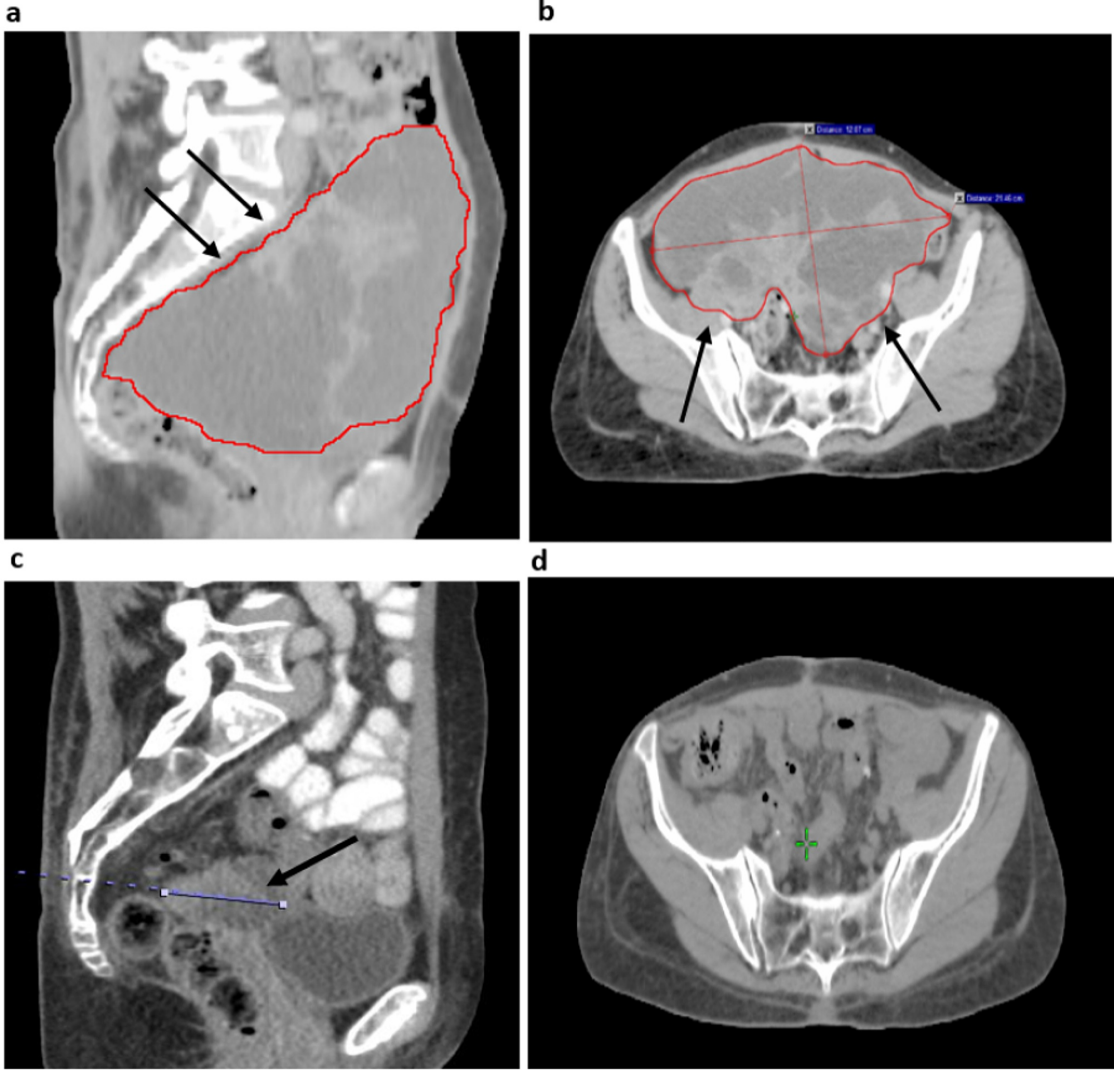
Recurrent intraperitoneal GCT before and after irradiation CT abdomen and pelvis demonstrating a large pelvic mass, outlined in red, in the (a) sagittal and (b) axial plane prior to starting radiation; images of the pelvis obtained three months after completing radiation therapy are depicted in (c) the sagittal view, where the maximum diameter is demarcated in blue, and (d) the axial view where the mass is no longer visible at this position. GCT: Granulosa cell tumor
